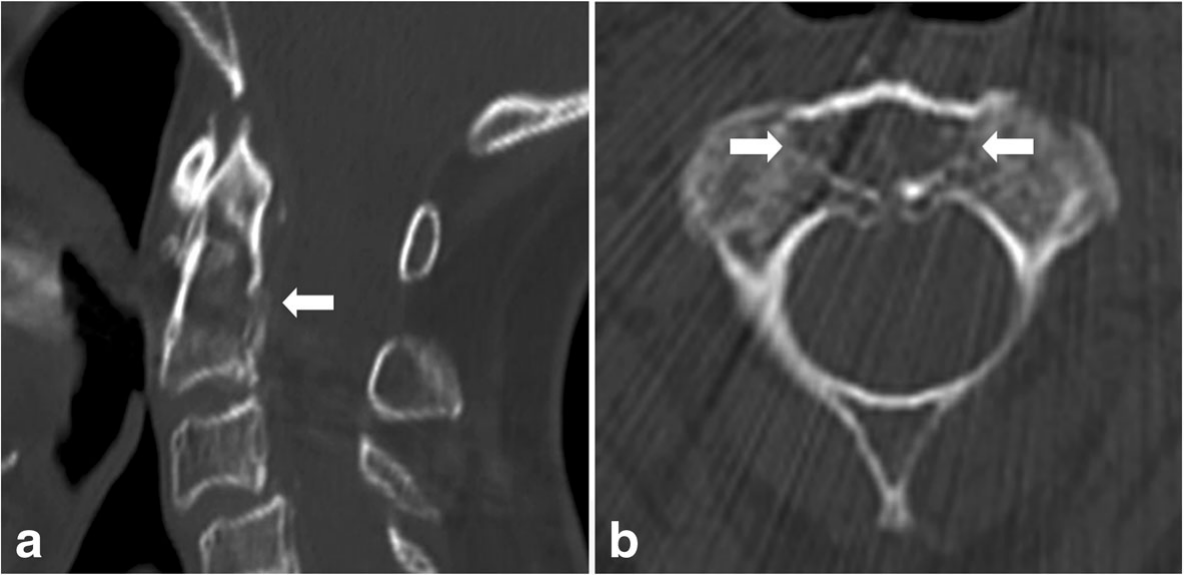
CT-scan in lateral (a) and axial view (b) of patient no. 2. A large osteolytic lesion in C2 (14 mm × 11 mm × 16 mm) is seen in the middle of the base and partially in the area of the odontoid process (arrows) (Case 2)
